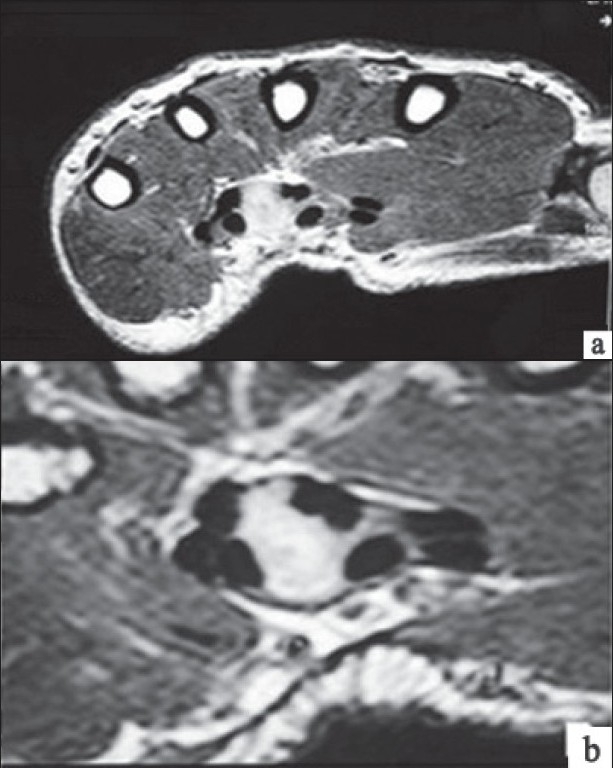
MRI wrist joint showing compression of median nerve by cysticercosis
